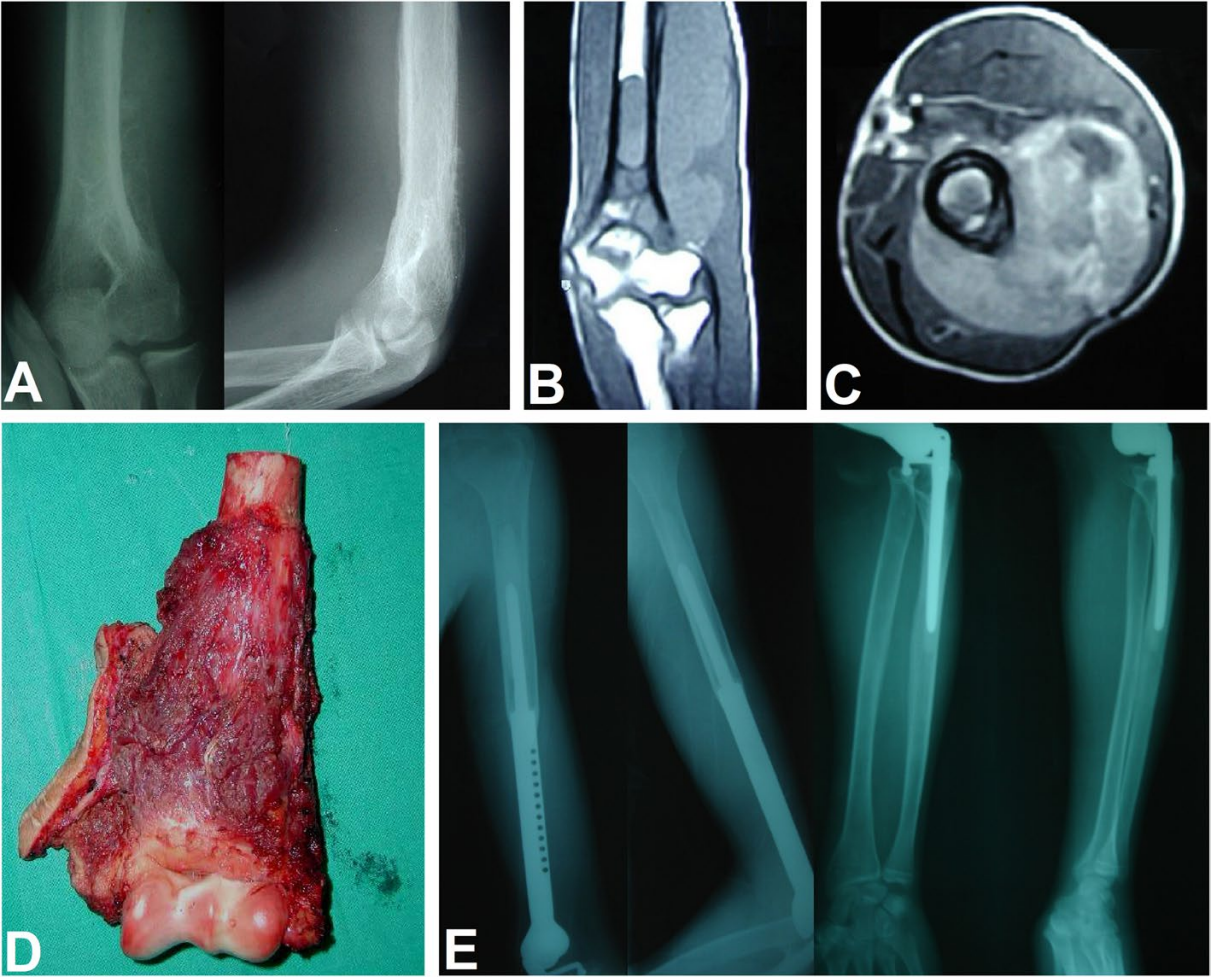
A 19-year-old male patient with Ewing's sarcoma of the distal humerus treated with wide resection and reconstruction by modular endoprosthesis. A Preoperative X-rays anteroposterior and lateral views. B Preoperative MRI coronal view. C Preoperative MRI axial view. D Intraoperative photograph of the resected distal humerus. E Six-year follow-up X-rays anteroposterior and lateral views
